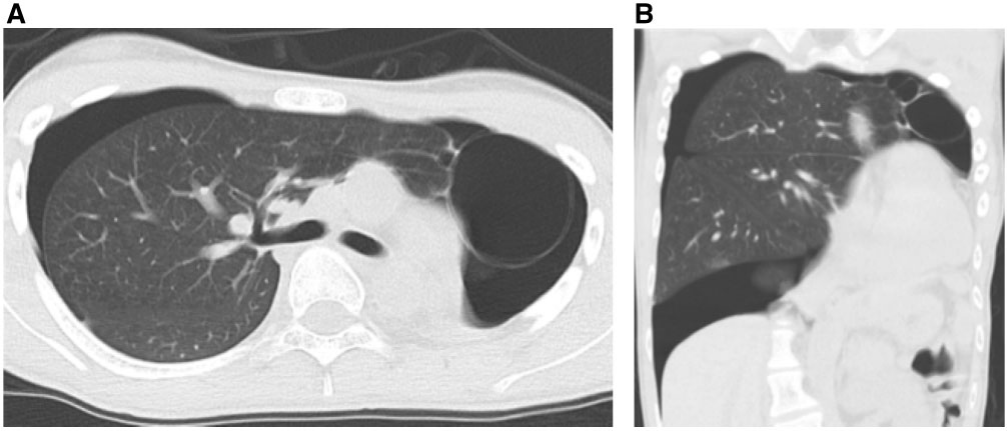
Chest computed tomography showed right pneumothorax after left pneumonectomy. Note that apical bullae located in the left atrophic chest cavity due to the overextension of the right pleural cavity beyond the upper mediastinum.
